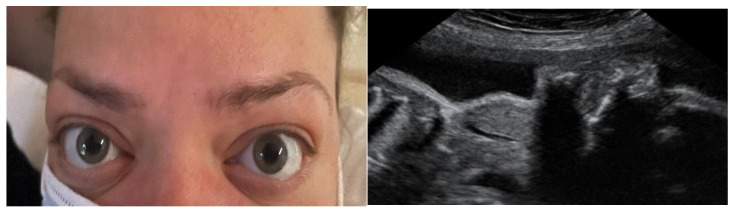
Orbithopathy in a pregnant woman with Graves’ disease (left). The fetus was diagnosed with goiter by ultrasound (right).
